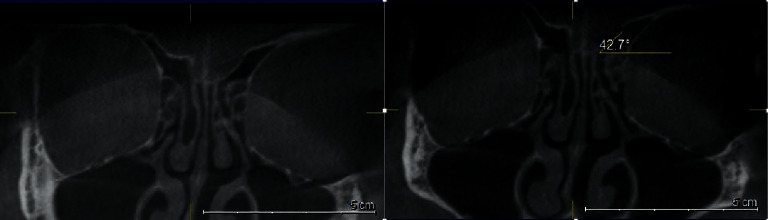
Coronal plan of CBCT demonstrating type III Gera classification on the left side.
